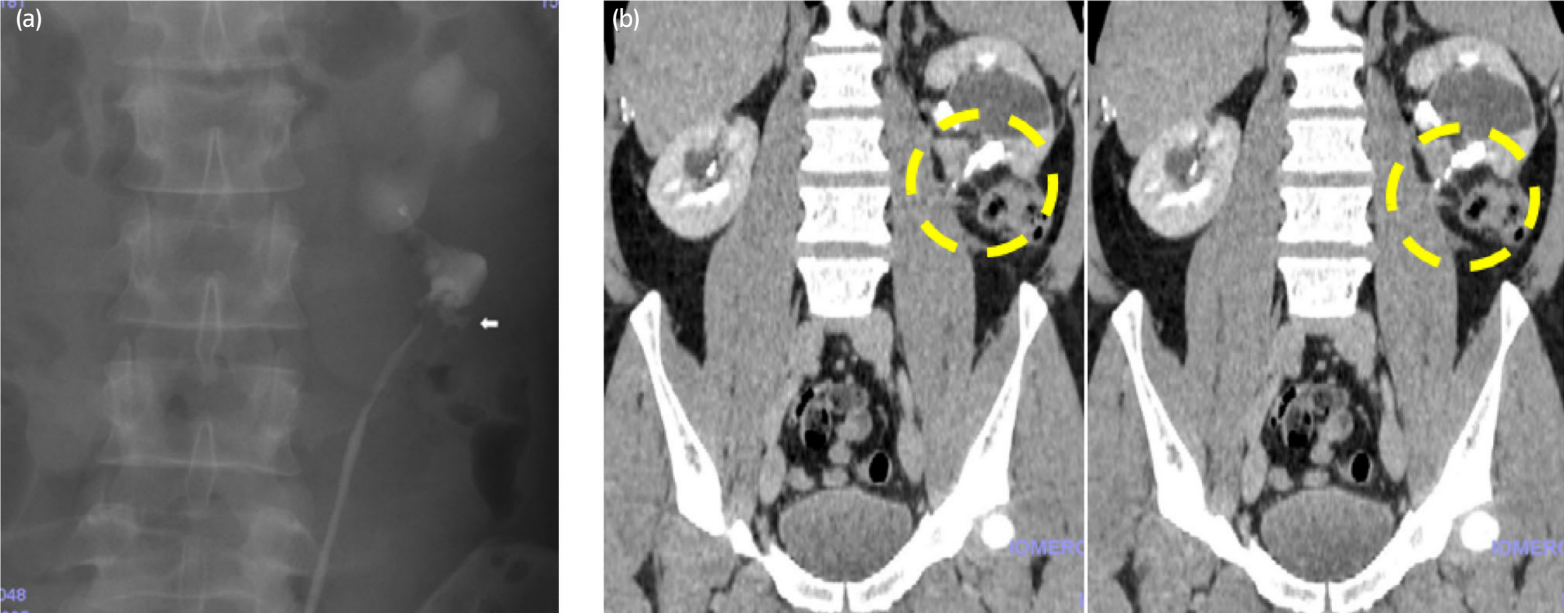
(a) Postoperative retrograde pyelogram at 1 month shows free flow of contrast medium into the renal collecting system and a leak from the site of anastomosis (arrow). (b) Postoperative contrast‐enhanced CT (CT‐KUB) at 2 months shows patency of the anastomosis (dotted circle) with no leakage.
